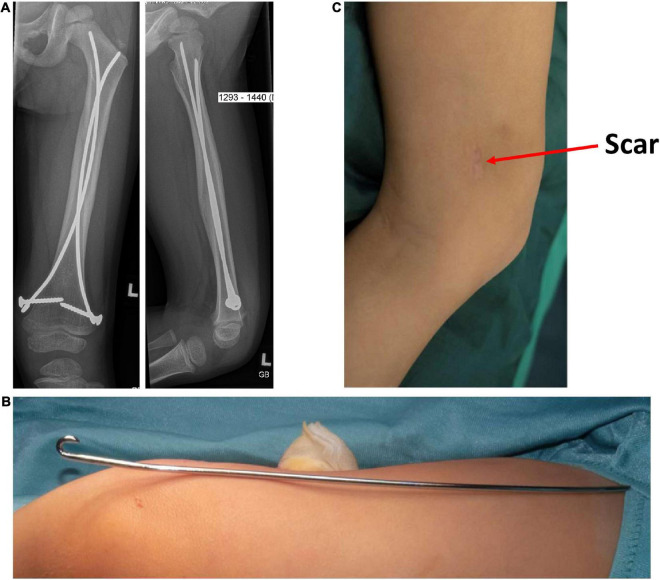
Surgical treatment: Postoperative radiographs are shown to the parents including pictures of the elastic, stable intramedullary nail (A,B) and the scar formation marked with an arrow (C).
